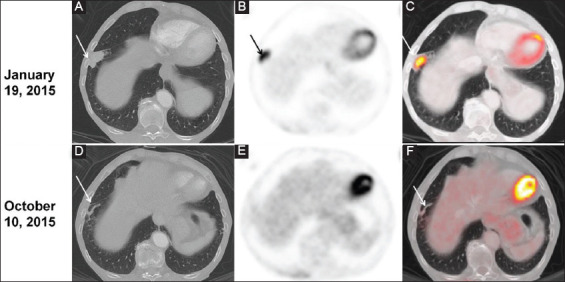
66-year-old woman, smoker, with severe COPD, and incidental finding of lower lobe nodule on chest X-ray. Axial 18F-fluorodeoxyglucose (18F-FDG) positron emission tomography/computed tomography (PET/CT) images (A, B, and C) in January 2015 showed a solid lobulated lung nodule with FDG uptake (SUVmax 12.9) (arrows), suspicious for primary pulmonary neoplasm. As the patient is not operable, she was treated with stereotactic body radiation therapy, finishing the treatment in June 2015. A 18F-FDG PET/CT in October 2015 (D, E and F) showed a linear scar without FDG uptake.
